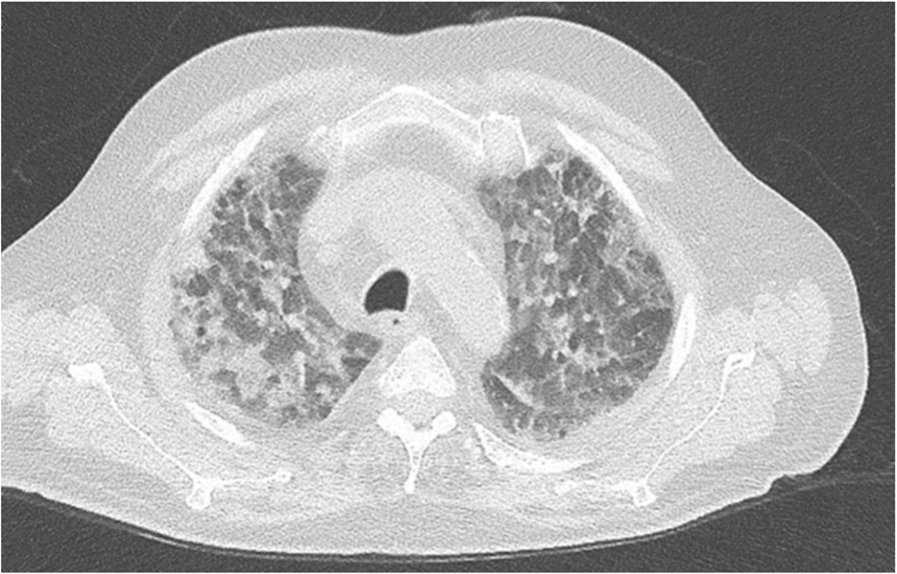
CT scan with bilateral pneumonic infiltrates
